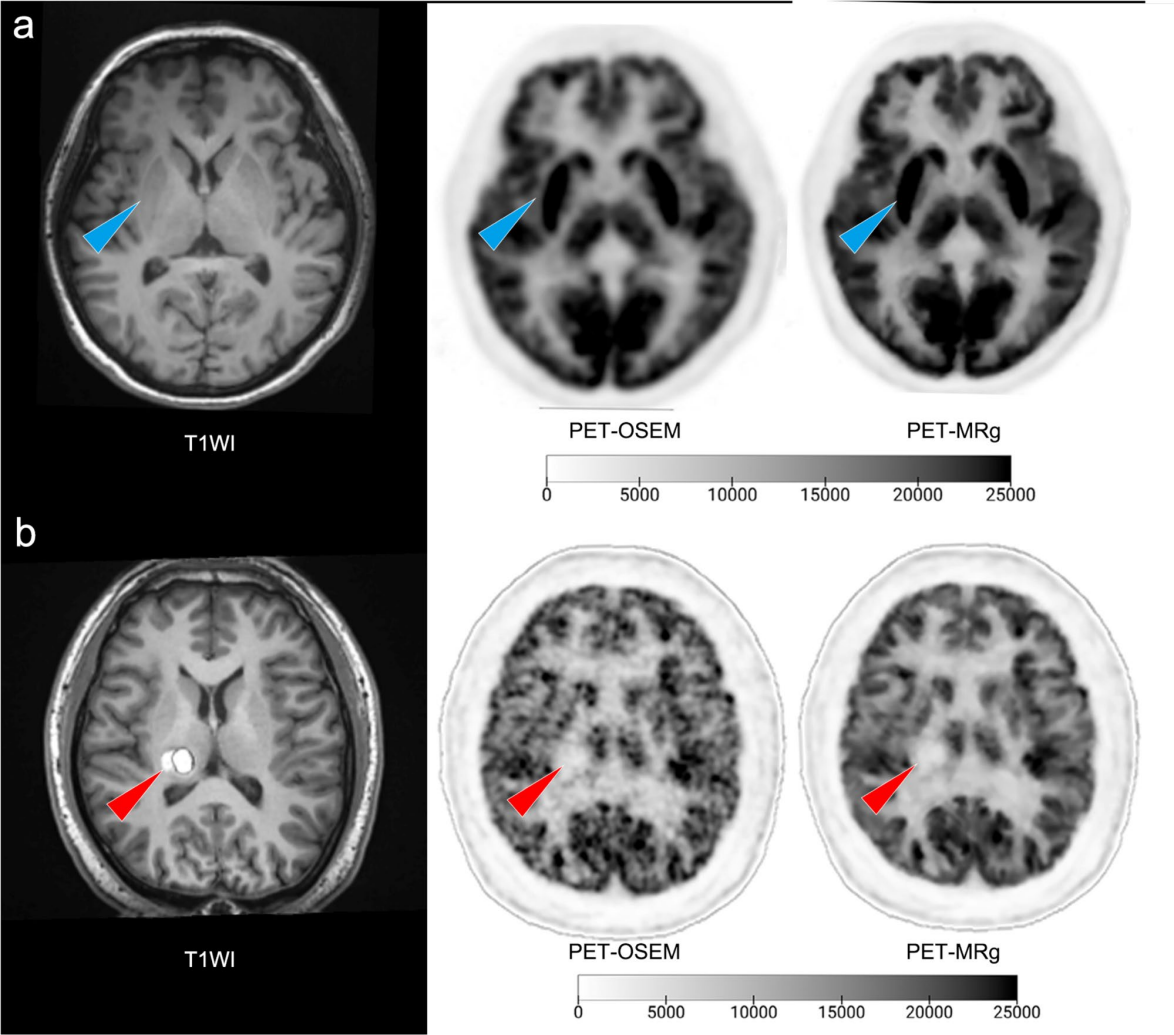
Example images of a patient with PD and a patient with cavernous angioma. (a) Shows the MR T1WI, PET‐OSEM, and PET‐MRg images of a 77‐year‐old male patient with Parkinson's disease visual analysis indicates that the PET‐MRg image is clearer than the PET‐OSEM images, with more uniform FDG metabolism and better‐defined boundaries in the putamina (blue arrow). (b) Depicts the MR T1WI, PET‐OSEM, and PET‐MRg images of a 45‐year‐old male patient with cavernous angioma. The PET‐MRg image depicts reduced metabolism in the lesion (red arrow) compared to normal brain white matter with a more precise identification of the lesion boundaries compared to PET‐OSEM. FDG, fluorodeoxyglucose; MR, magnetic resonance; MRg, magnetic resonance‐guided PET reconstruction; OSEM, ordered subset expectation maximization; PD, Parkinson's disease; PET, positron emission tomography; T1WI, T1 weighted image.
